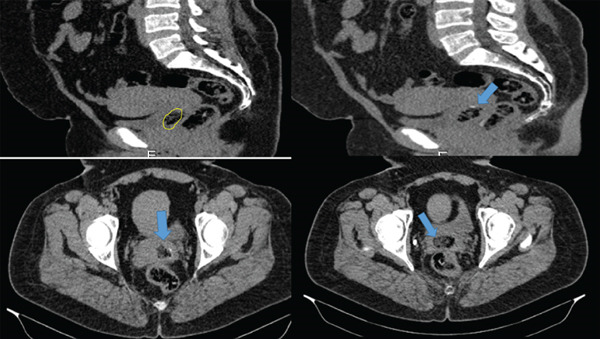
Computed tomography (CT) images reconstructed in sagittal and axial planes.
